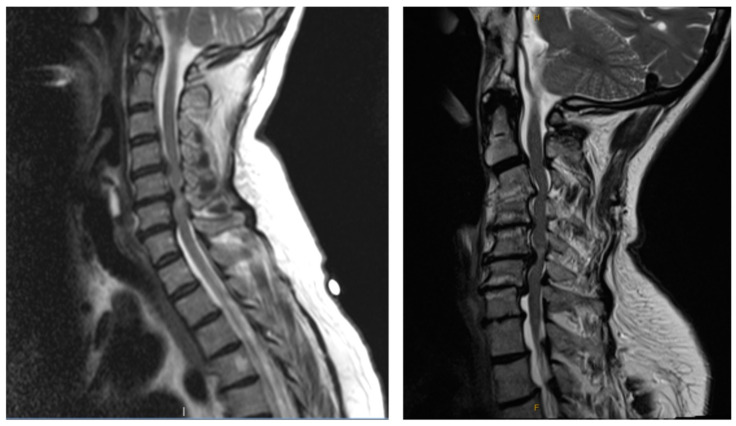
T2-weighted MRI demonstrating a patient with congenital stenosis and multi-level stenosis from disc herniations (left) and a patient with congenital stenosis and multi-level stenosis from disc degeneration and kyphotic deformity (right).
